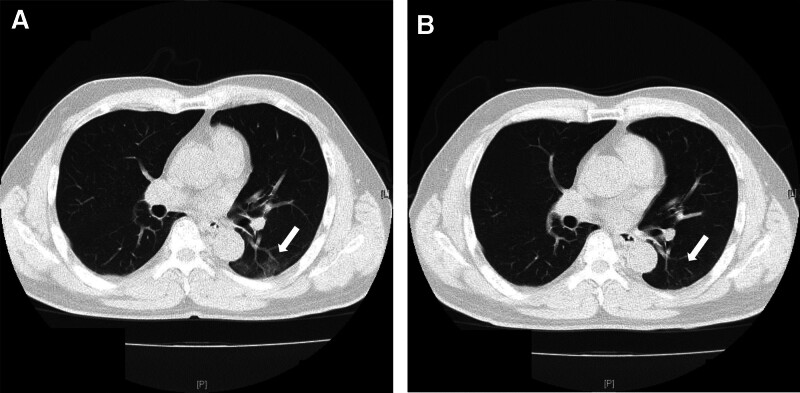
LDCT image of pulmonary infection case taken for 1-yr period. (A)LDCT showing inflammatory change in the left lower lung field (white arrow). (B)LDCT of this case 1 yr after the first examination. This lesion finally improved spontaneously without any medication (white arrow). LDCT = low-dose computed tomography.
